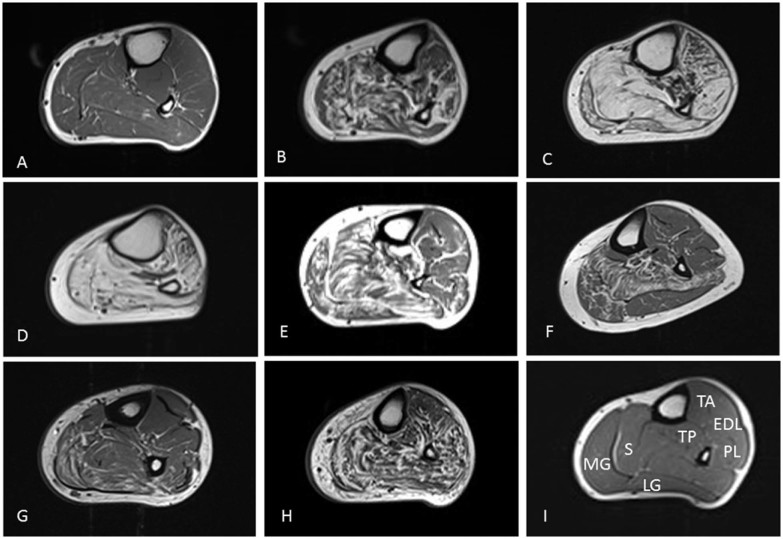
T1 weighted axial MRI images of the left lower limb muscles at mid-calf level in patients with mutations in *HSPB1*. Denervated muscle shows fat infiltration (white). A = patient 2 (Mercuri score = 6), B = patient 7 (41), C = patient 8 (48), D = patient 11 (46), E = patient 12 (34), F = patient 13 (i) (22), G = patient 13 (ii) (20), H = patient 13 (iv) (41), I = Healthy volunteer. Abbreviations: MG = Medial Head of Gastrocnemius, LG = Lateral Head of Gastrocnemius, S = Soleus, TP = Tibialis Posterior, TA = Tibialis Anterior, EDL = Extensor Digitorum Longus, PL = Peroneus Longus.
